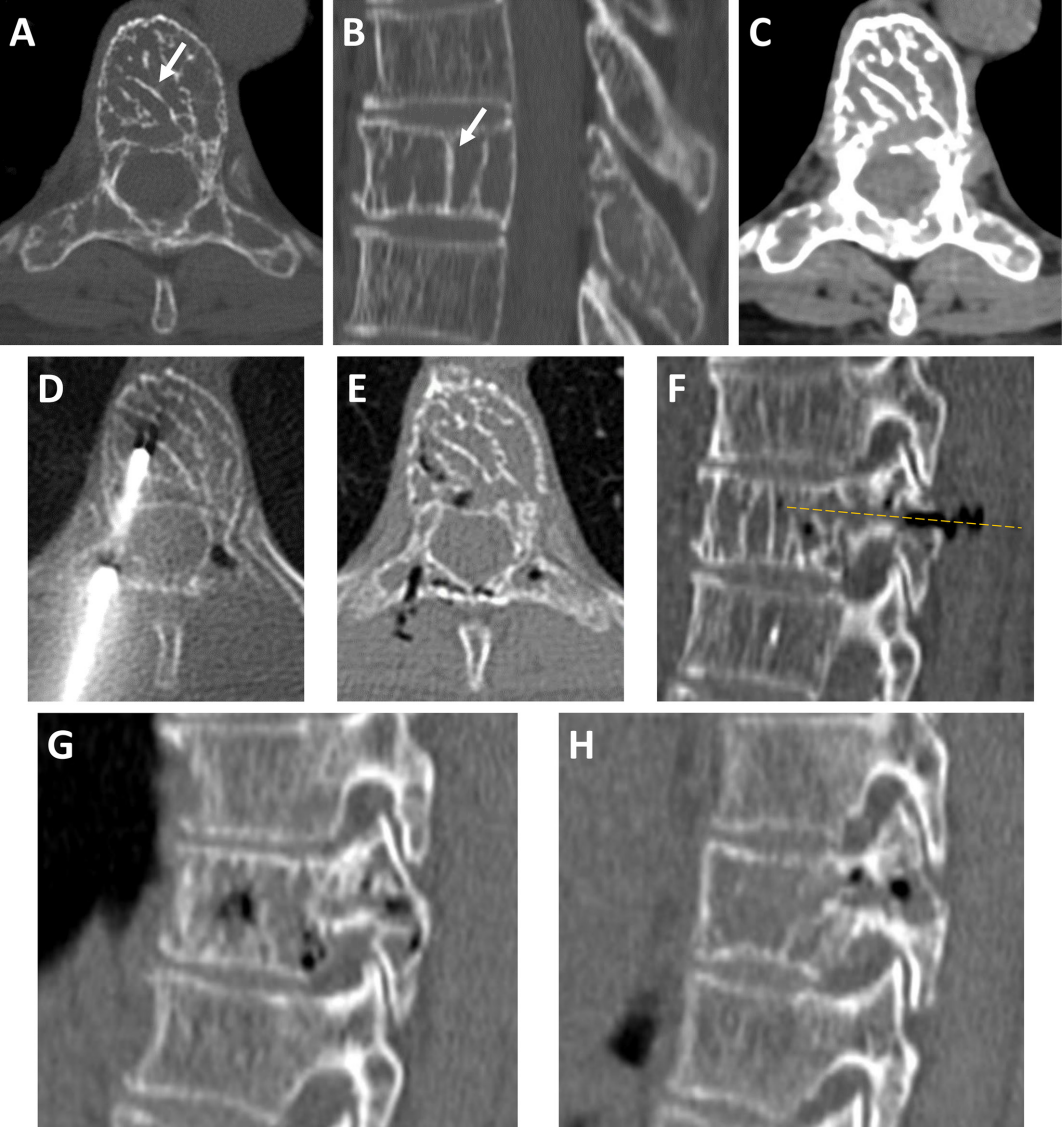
CT-scan of an osteolytic lesion of the T8 vertebra, composed of thickened vertical trabeculae *[arrows in axial slice (A) with sagittal reconstruction (B) in bone window*]. The lesion involved the vertebral body and the posterior arch, with some degree of hyperostosis of the whole vertebra [enlargement of the anteroposterior diameter in *(B*)]. There was only mild paravertebral soft tissue extension (*C: axial slice in soft tissue window*). After CT-guided biopsy through the right pedicle (*D*), gas bubbles have formed along the biopsy path and remotely inside the lesion (*E*). Sagittal reconstruction better shows the biopsy path through the pedicle (*dotted yellow line*), partially filled with gas bubbles (*F*). Note the presence of gas bubbles in both pedicles after biopsy (*G: right pedicle in sagittal slice; H: left pedicle in sagittal slice*).
